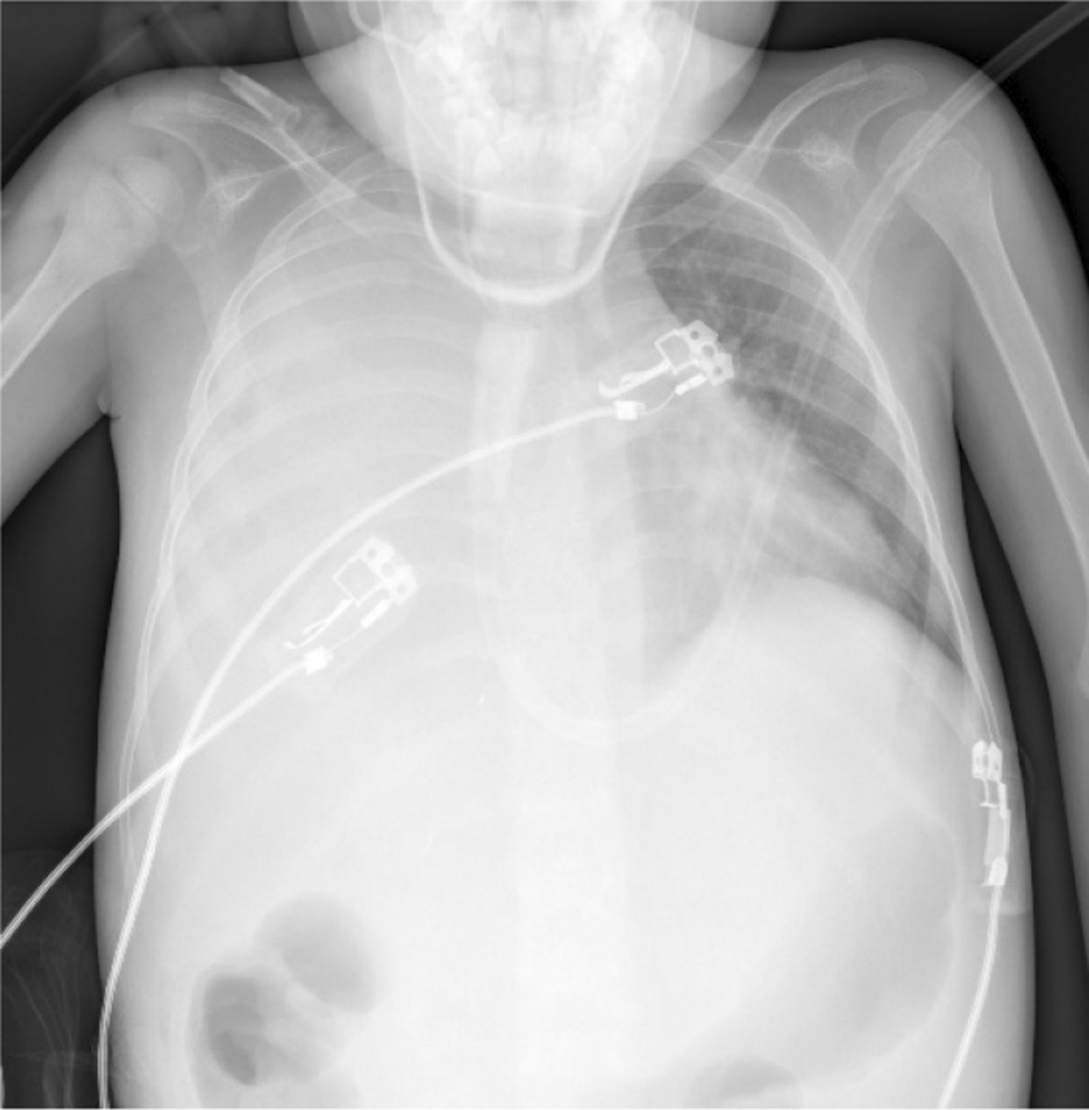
Chest roentgenogram demonstrating massive right side pleural effusion, due to biliopleural Fistula.
